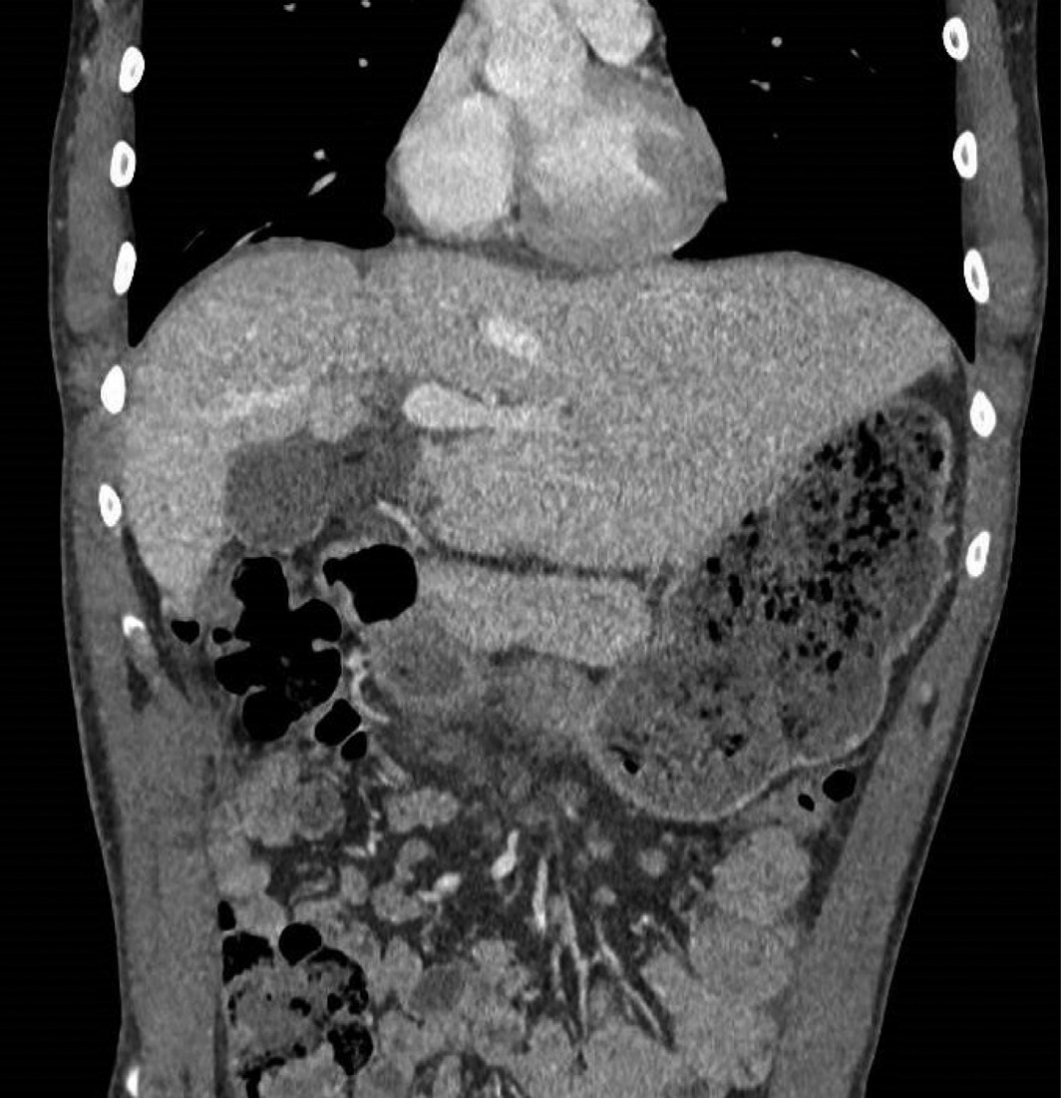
Thoracoabdominal CT scan at postoperative day 11 showing notable hypotrophy of the right liver.
